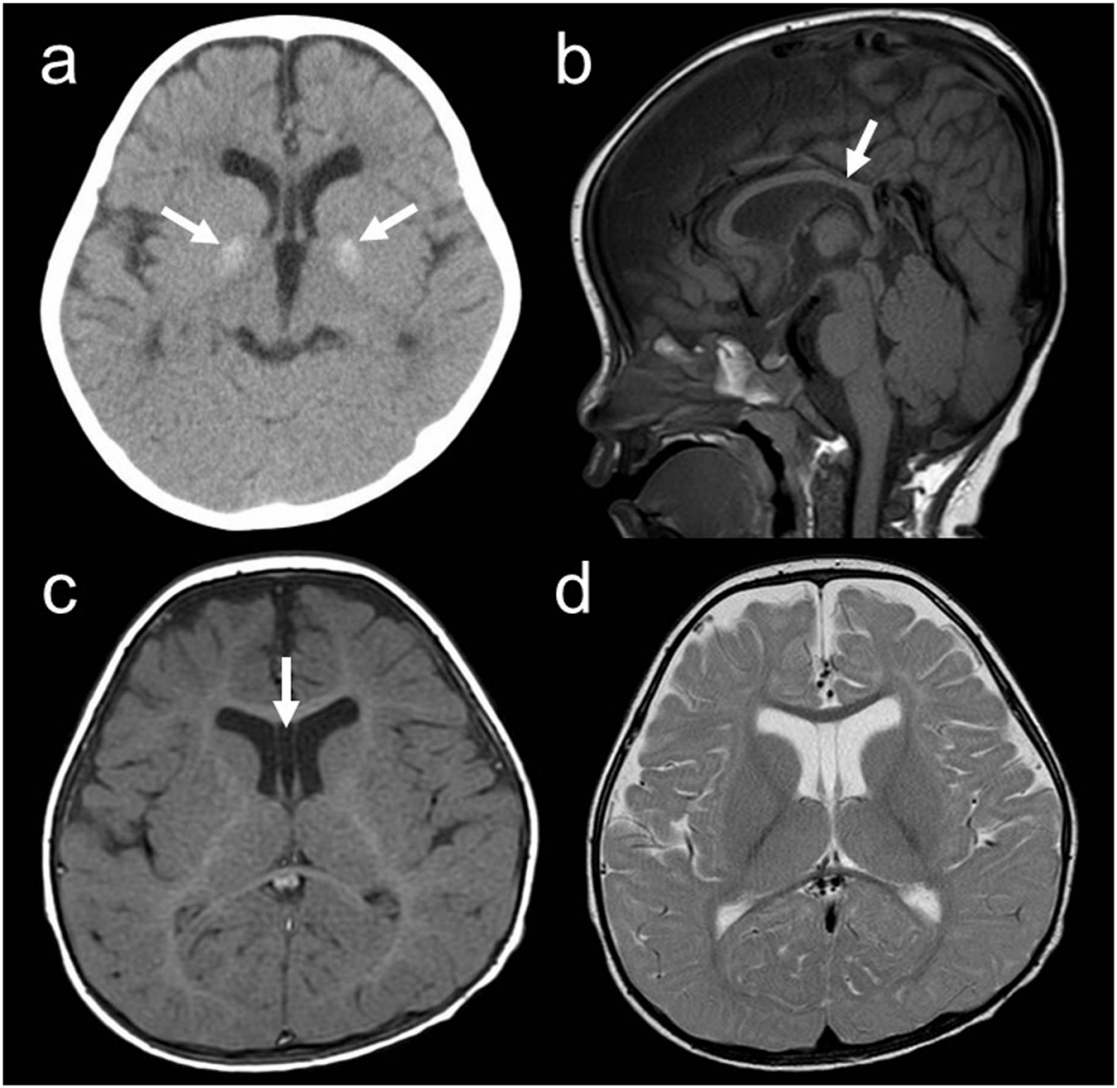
Radiological findings of the present patient. a Cranial computed tomography image. Calcification is shown in the bilateral basal ganglia (arrows). b Sagittal image of brain magnetic resonance imaging, indicating thinning of the corpus callosum (arrow). c, d T1- and T2-weighted brain magnetic resonance axial images indicating abnormalities in several regions, including the cavus septum pellucidum (arrow).
